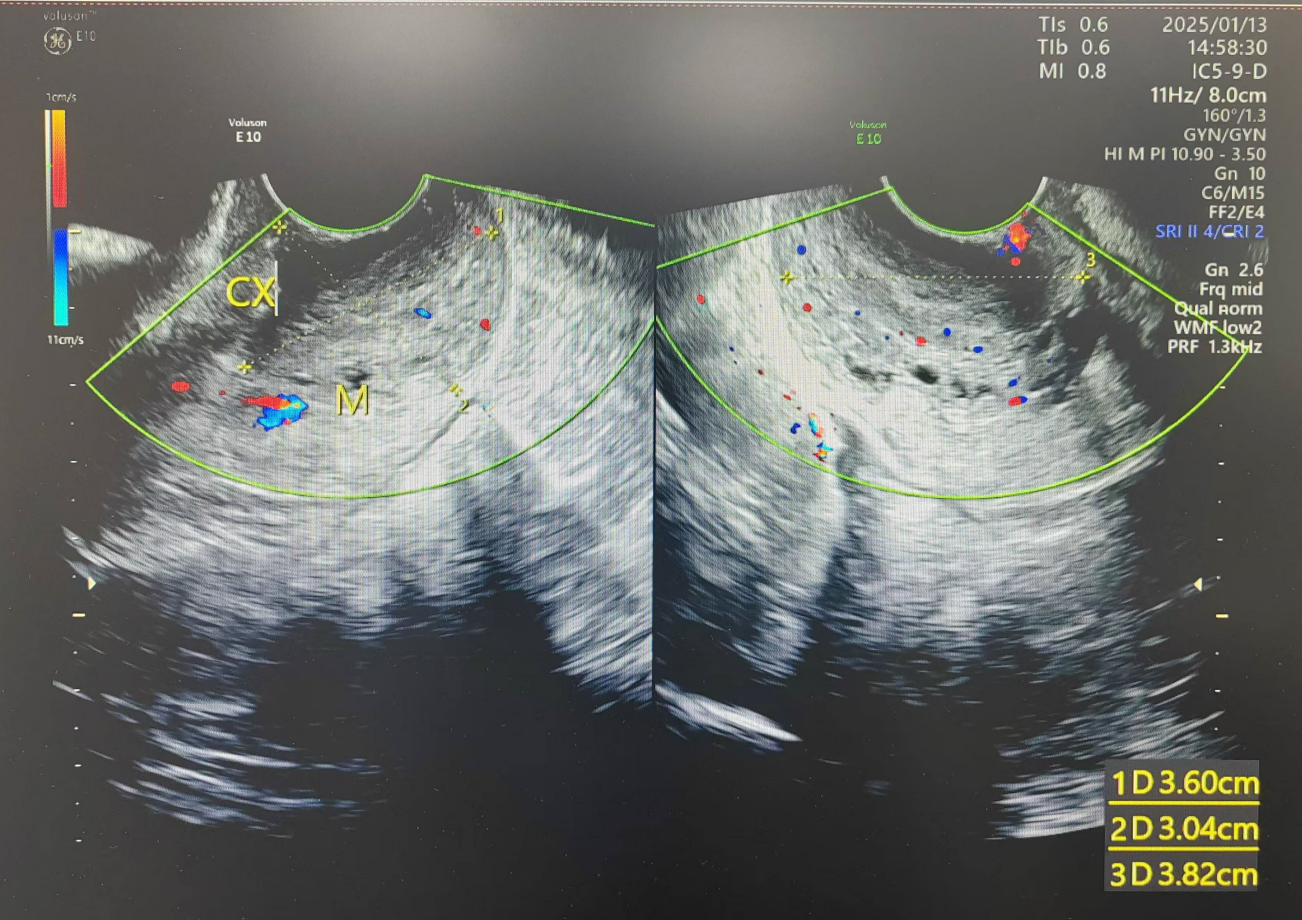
Ultrasonography demonstrated an area of heterogeneous echoes at the cervix with unclear boundaries, measuring 3.8×3.6×3.0 cm.
